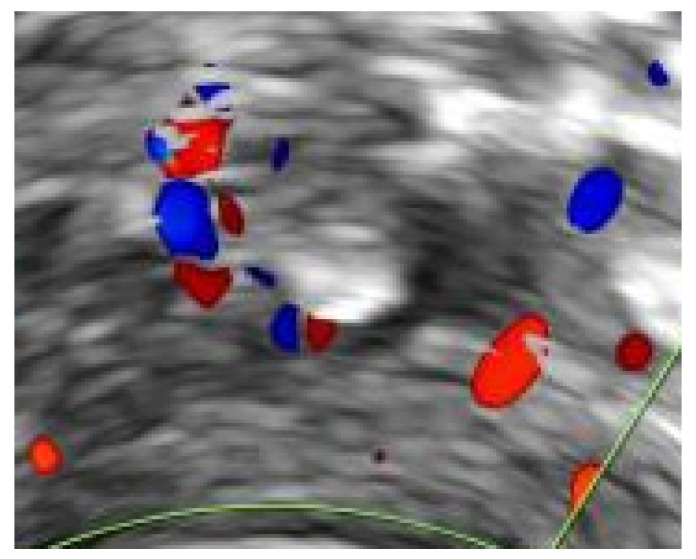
Transvaginal image of uterine vein phlebolith with colour Doppler.
